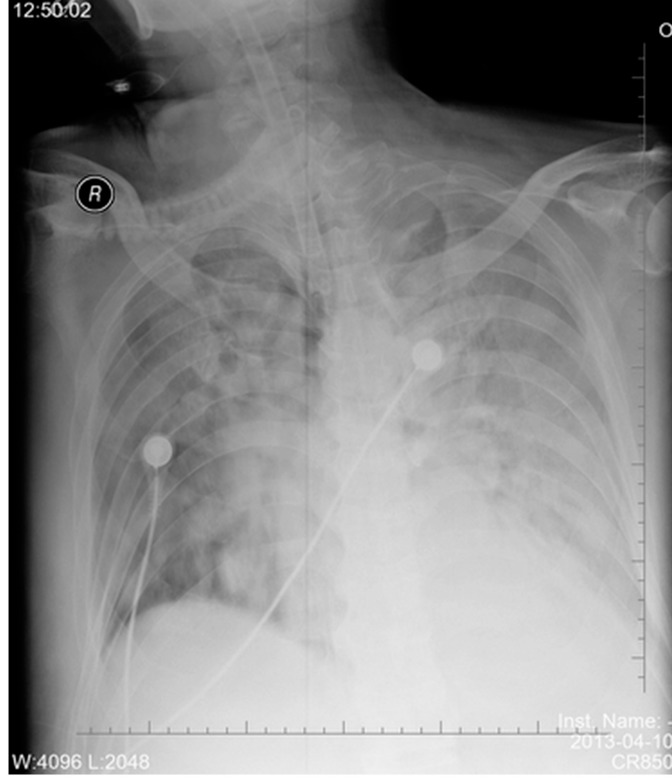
On April 10^th^ 2013, the chest radiograph showed the two pulmonary diffuse ground glass opacities, with a degree of fusion into nodules.
